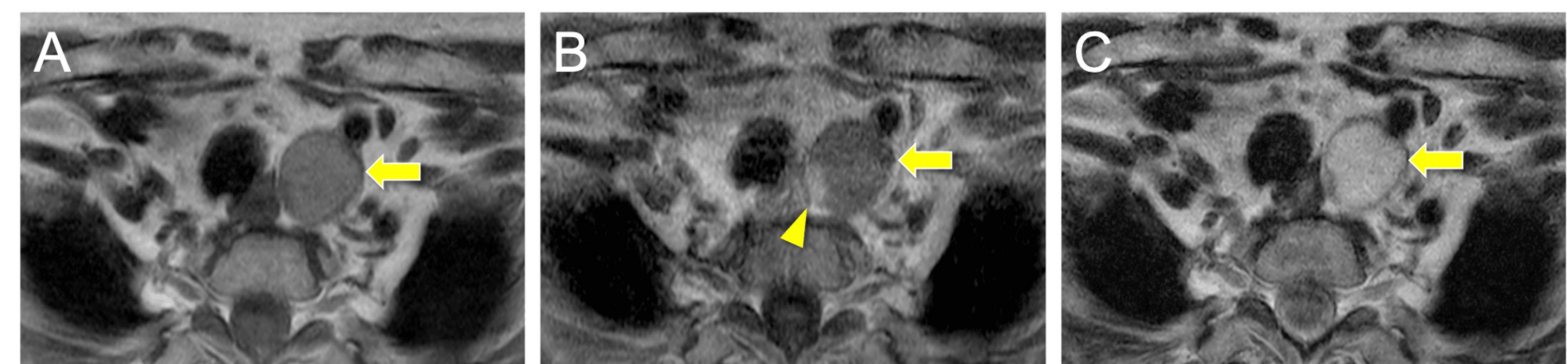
Preoperative MRI (A) The tumor showed hypointensity on T1-weighted MRI. (B) On gadolinium-enhanced MRI, only a small area corresponding to the CT-enhanced region showed enhancement (arrowhead). (C) The tumor showed hyperintensity on T2-weighted MRI images.
